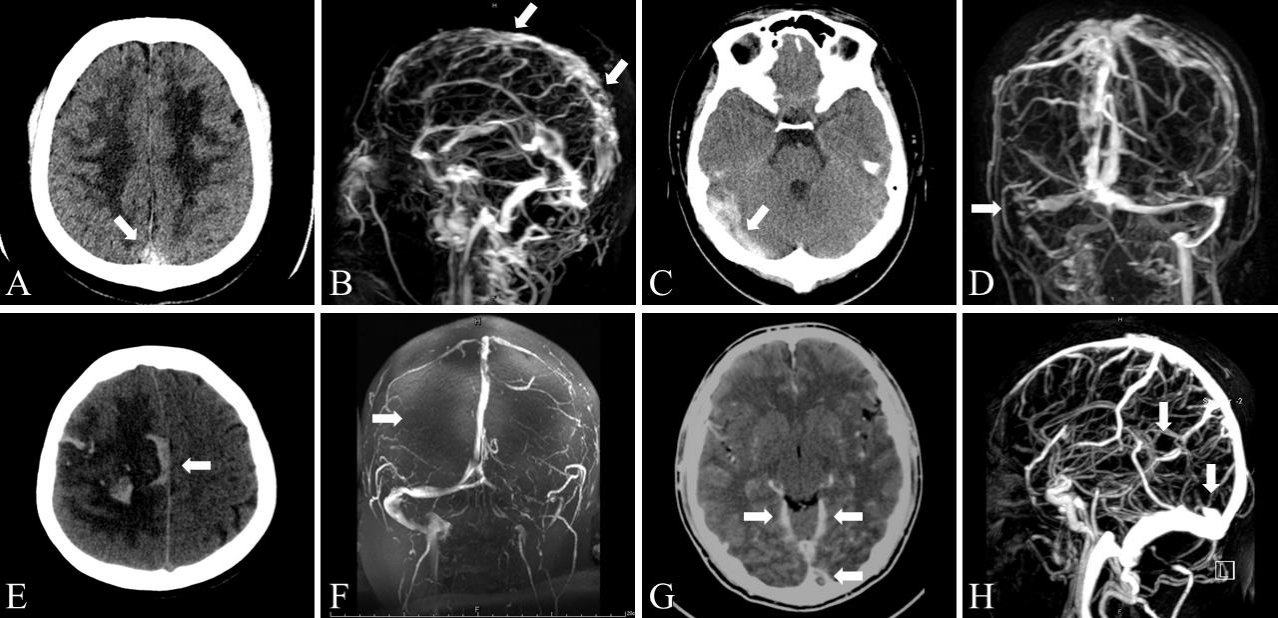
Imaging findings using CT and MRV in patients with CVT. (A-B) Superior sagittal sinus thrombosis. Axial CT demonstrated hyperintense signal intensity of the superior sagittal sinus (A). Sagittal CE-MRV confirmed thrombosis in the superior sagittal sinus (B). (C-D) Right transverse sinus and sigmoid sinus thrombosis. Axial CT demonstrated hyperintense signal intensity of the right transverse sinus (C). MRV confirmed thrombosis in the right transverse sinus and sigmoid sinus (D). (E-F) Cortical venous thrombosis. Axial CT scan of a patient with cortical venous thrombosis showed a venous hemorrhagic infarct in the right parietal lobe (E). TOF-MRV showed disappearance of the right cortical veins. The white arrow indicates that this right cortical venous disappearance was caused by a thrombus (F). (G-H) Cerebral deep venous thrombosis. Hyperintense signal intensity of the straight sinus and bilateral deep cerebral veins was observed using axial CT (G). With sagittal CE-MRV, the straight sinus and bilateral deep cerebral veins were not observed. The white arrows indicate filling defects that were caused by the thrombus (H).
